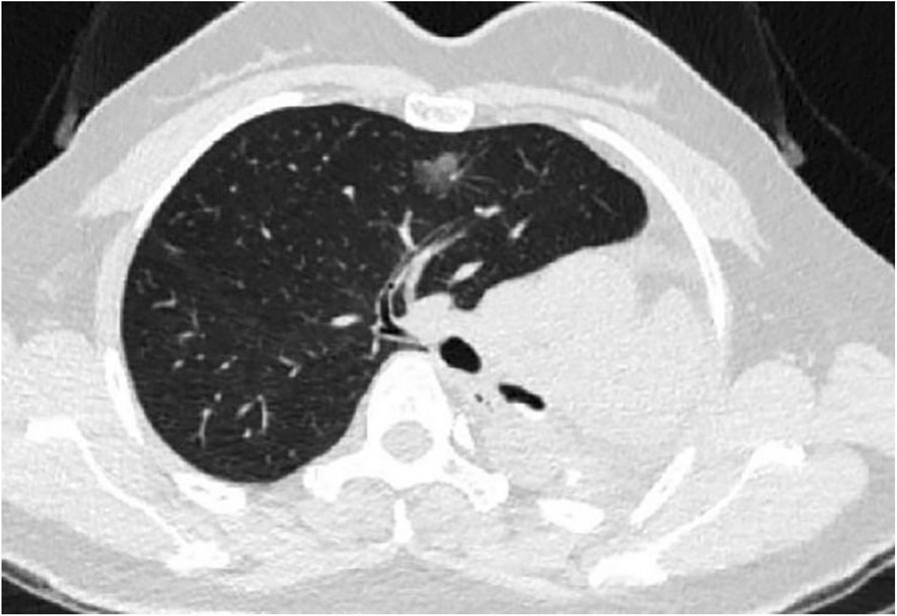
CT (computed tomography) scan of the right anterior GGO (ground glass opacity) and postpneumonectomy
